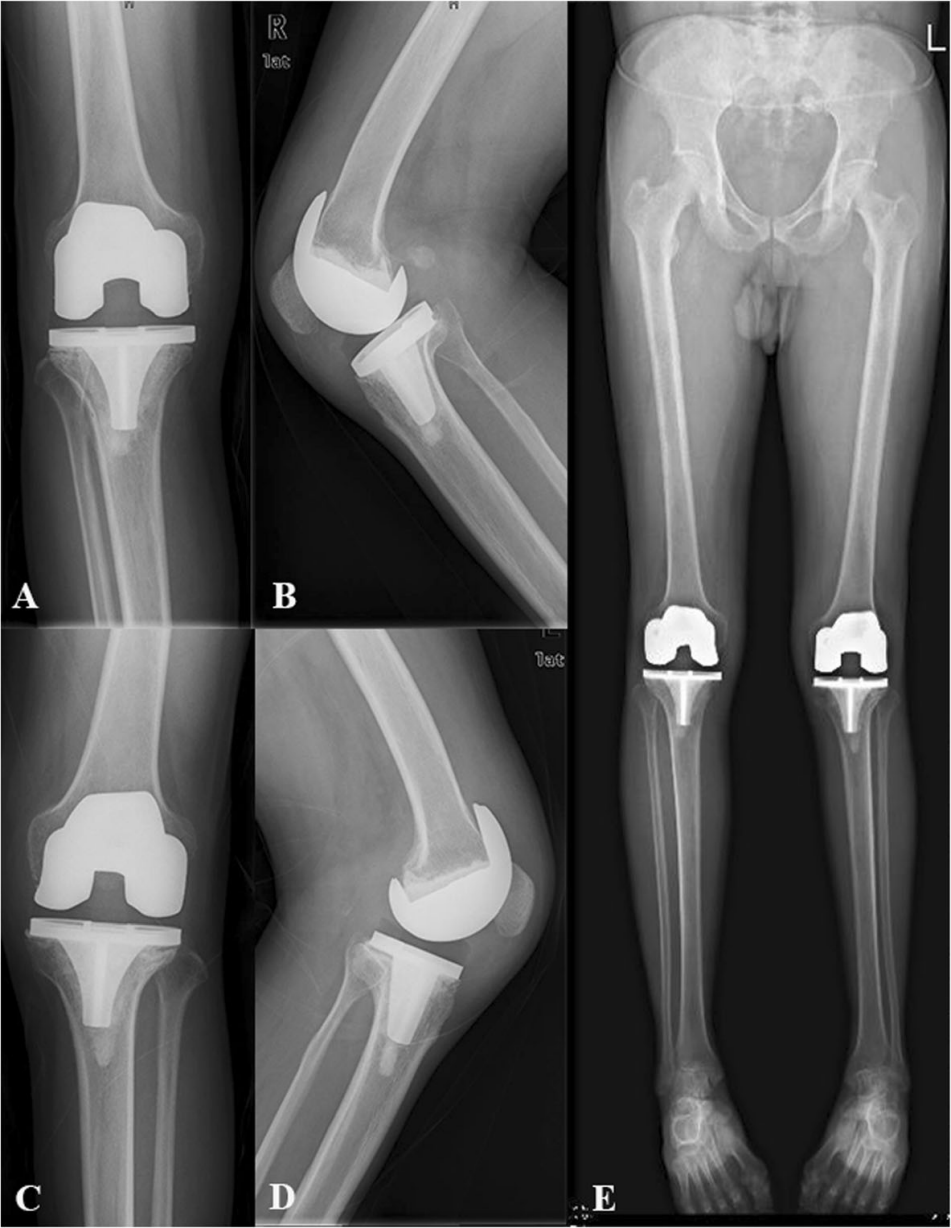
One year follow-up X-ray of simultaneous bilateral TKA. a AP view of right knee; b Lateral view of right knee; c Anteroposterior (AP) view of left knee; d Lateral view of left knee; e Full-length standing AP view. (No progressive radiolucent line > 2 mm in width)
